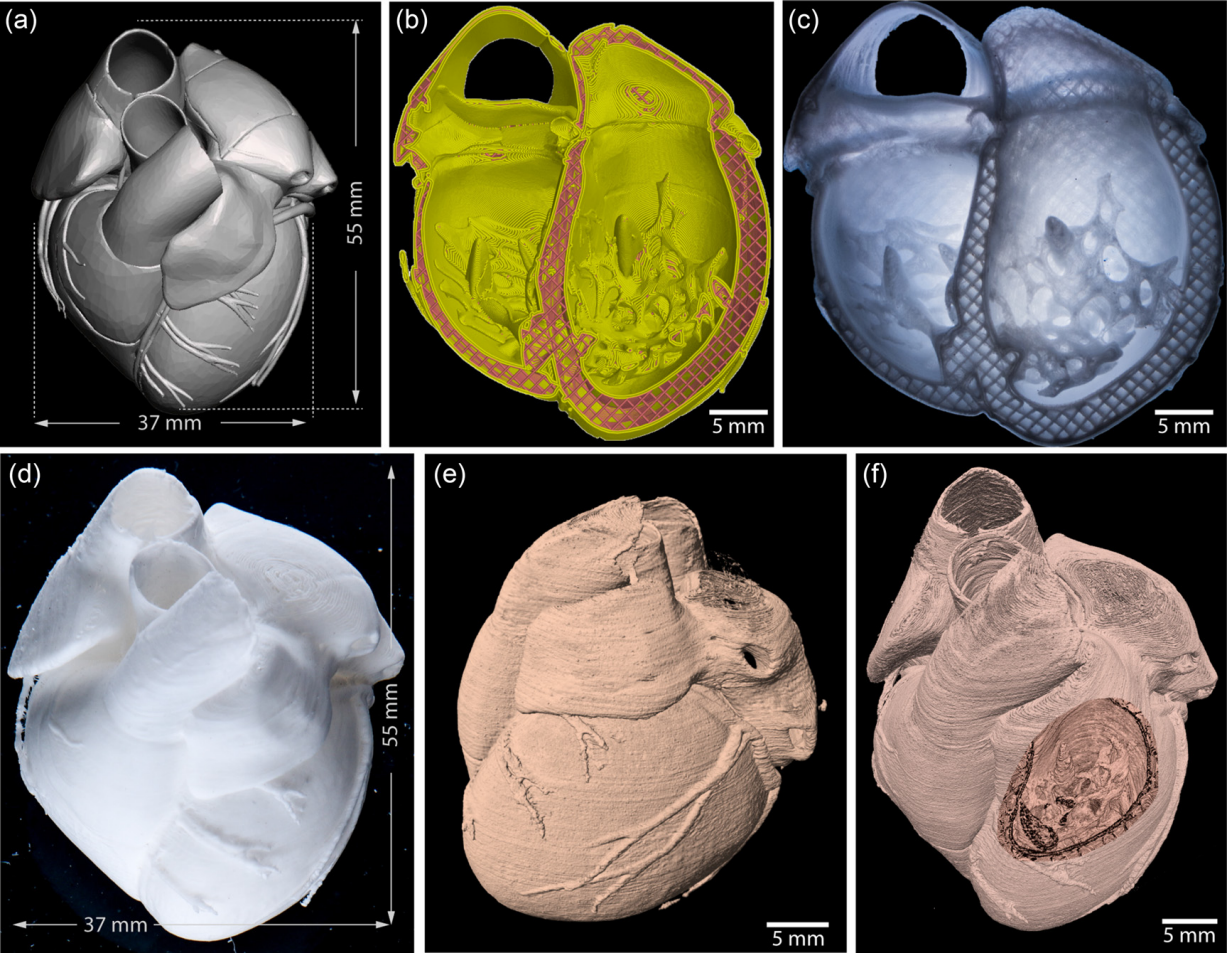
FRESH printing a patient-specific neonatal scale human heart from collagen I. (a) 3D STL model of a human heart scaled to the neonatal size adapted from Lee *et al.*^10^ (b) Visualization of the 3D print pathing for the modeled heart. (c) A half print of the heart revealing the internal complex geometry and the 3D printed infill pattern. (d) A full neonatal scale FRESH-printed heart from collagen I containing barium sulfate for subsequent *μ*CT imaging. (e) A 3D render of the *μ*CT of the FRESH-printed human heart. (f) A windowed view into the interior of the printed heart as viewed by *μ*CT data. These are original unpublished data related to work on collagen bioprinting reproduced with permission from Lee *et al.*, Science 365(6452), 482–487 (2019). Copyright 2019 American Association for the Advancement of Science.
